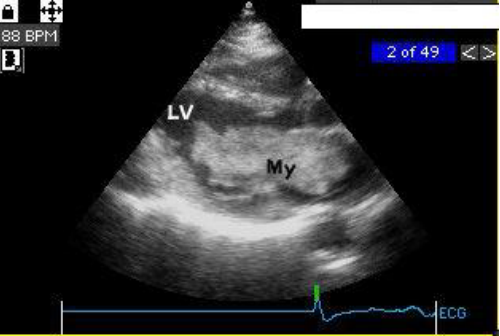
Echocardiography (45 months) long axis view. Myxoma in the left atrium prolapsing into the left ventricle. LV = left ventricle, My = myxoma
